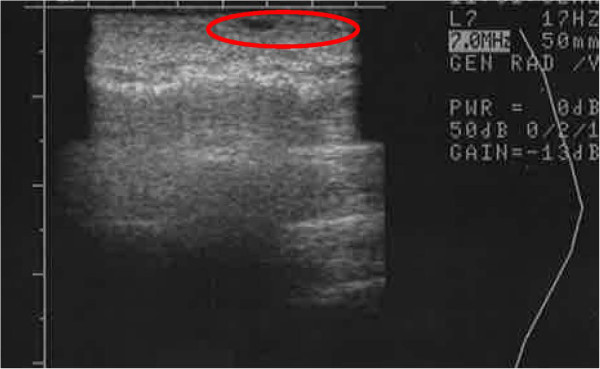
Ultrasound image of right hand (control) saggital view of thenar web space of a 69-year-old female 3 months post lipotransfer. Oil cysts seen in superficial dermis (red circle).
